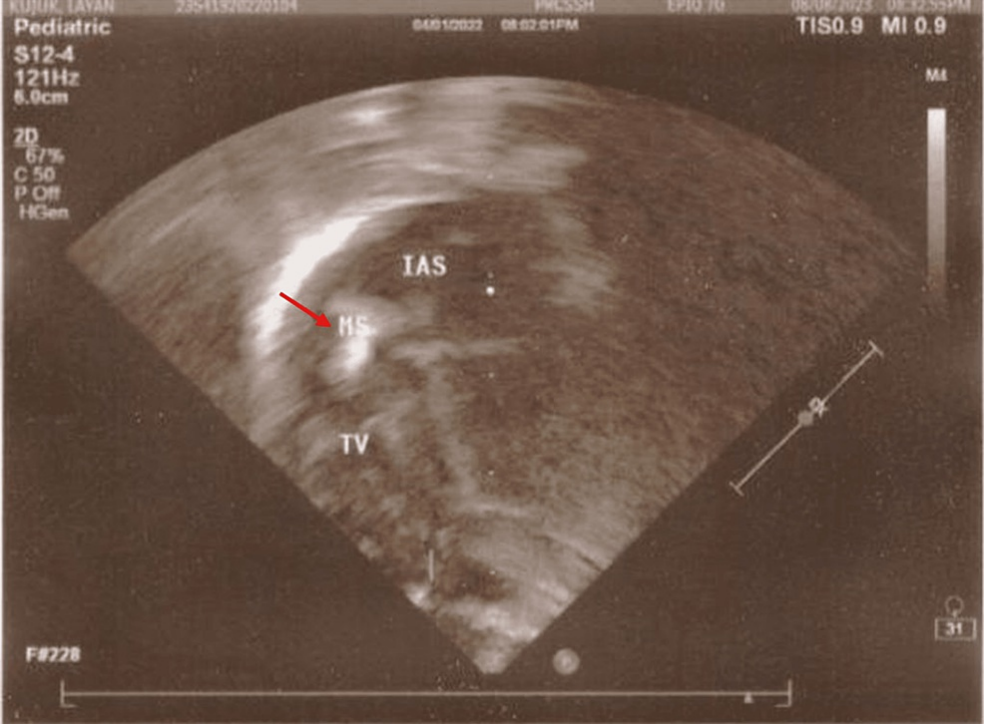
Echocardiogram depicting a substantial dynamic mass measuring 10x3 mm (red arrow) within the right atrium. MS, mass; IAS, interatrial septum; TV, tricuspid valve.
